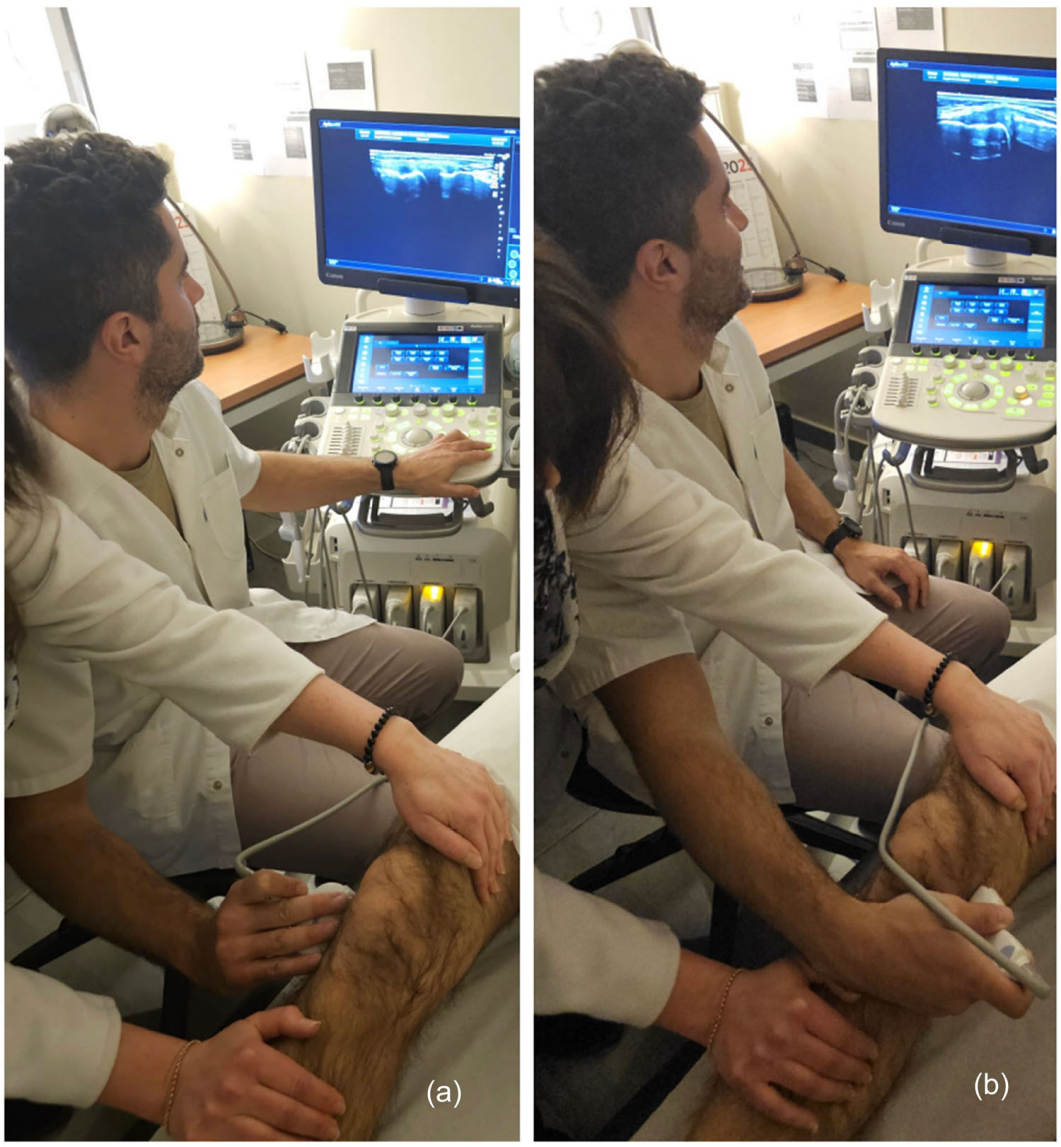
Ultrasound stress testing at 30° of flexion: (a) Lateral compartment in varus stress. (b) Medial compartment in valgus stress.
